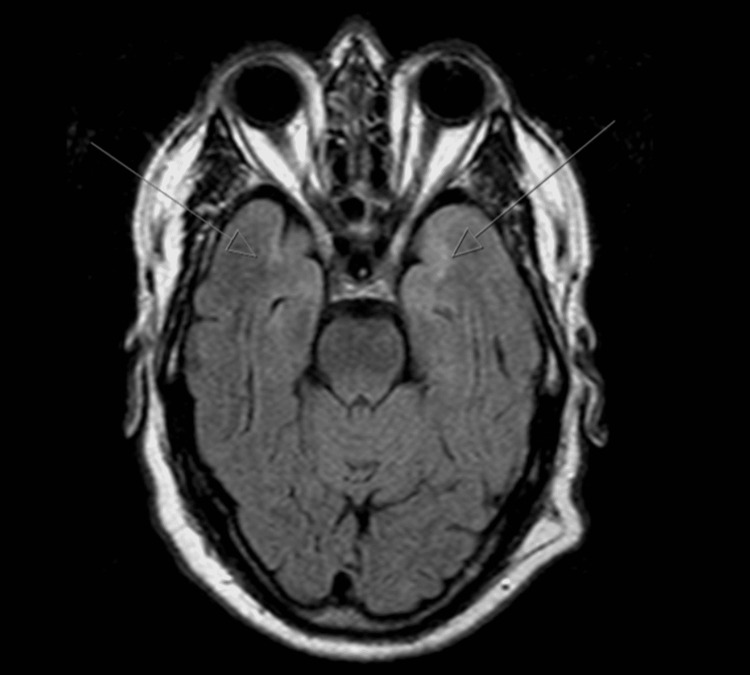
Brain MRI axial FLAIR showing hyperintensities in the anterior temporal lobe bilaterally FLAIR: fluid-attenuated inversion recovery
